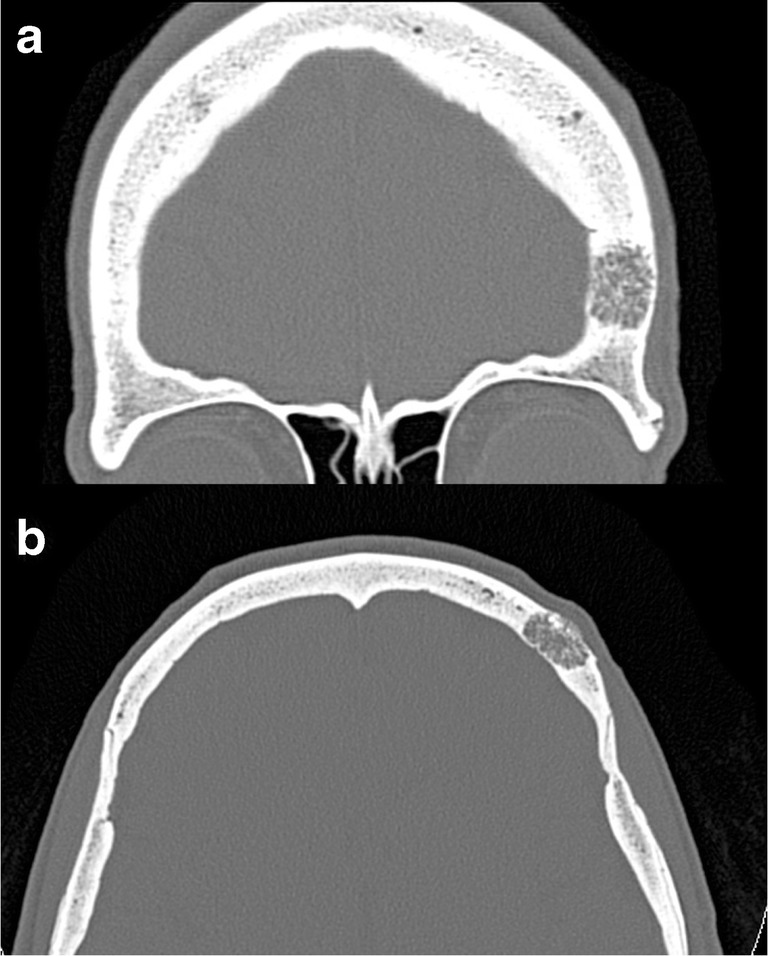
Intraosseous haemangioma (IH). Coronal (a) and axial (b) reformats of a CT scan with bone window indicate a left frontal expansive lesion with thin margins and intralesional spicules, radiating from a common centre, which erodes the outer layer of the skull, with relative sparing of the inner table
